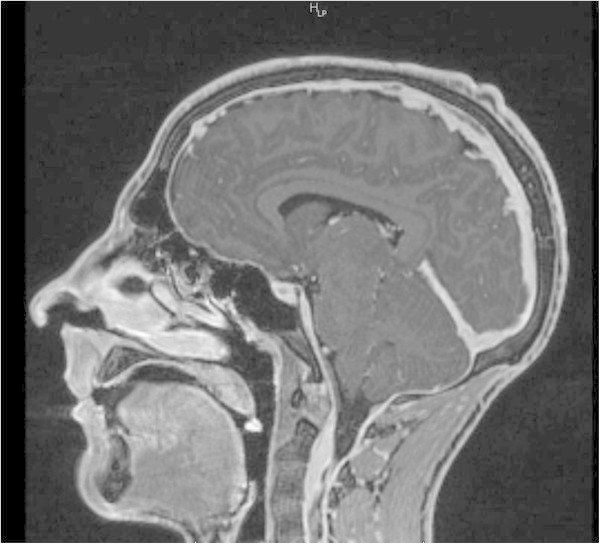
Case II: sagittal- weighted T1 MRI with contrast medium demonstrating dural enhancement and descent of the cerebellar tonsil.
